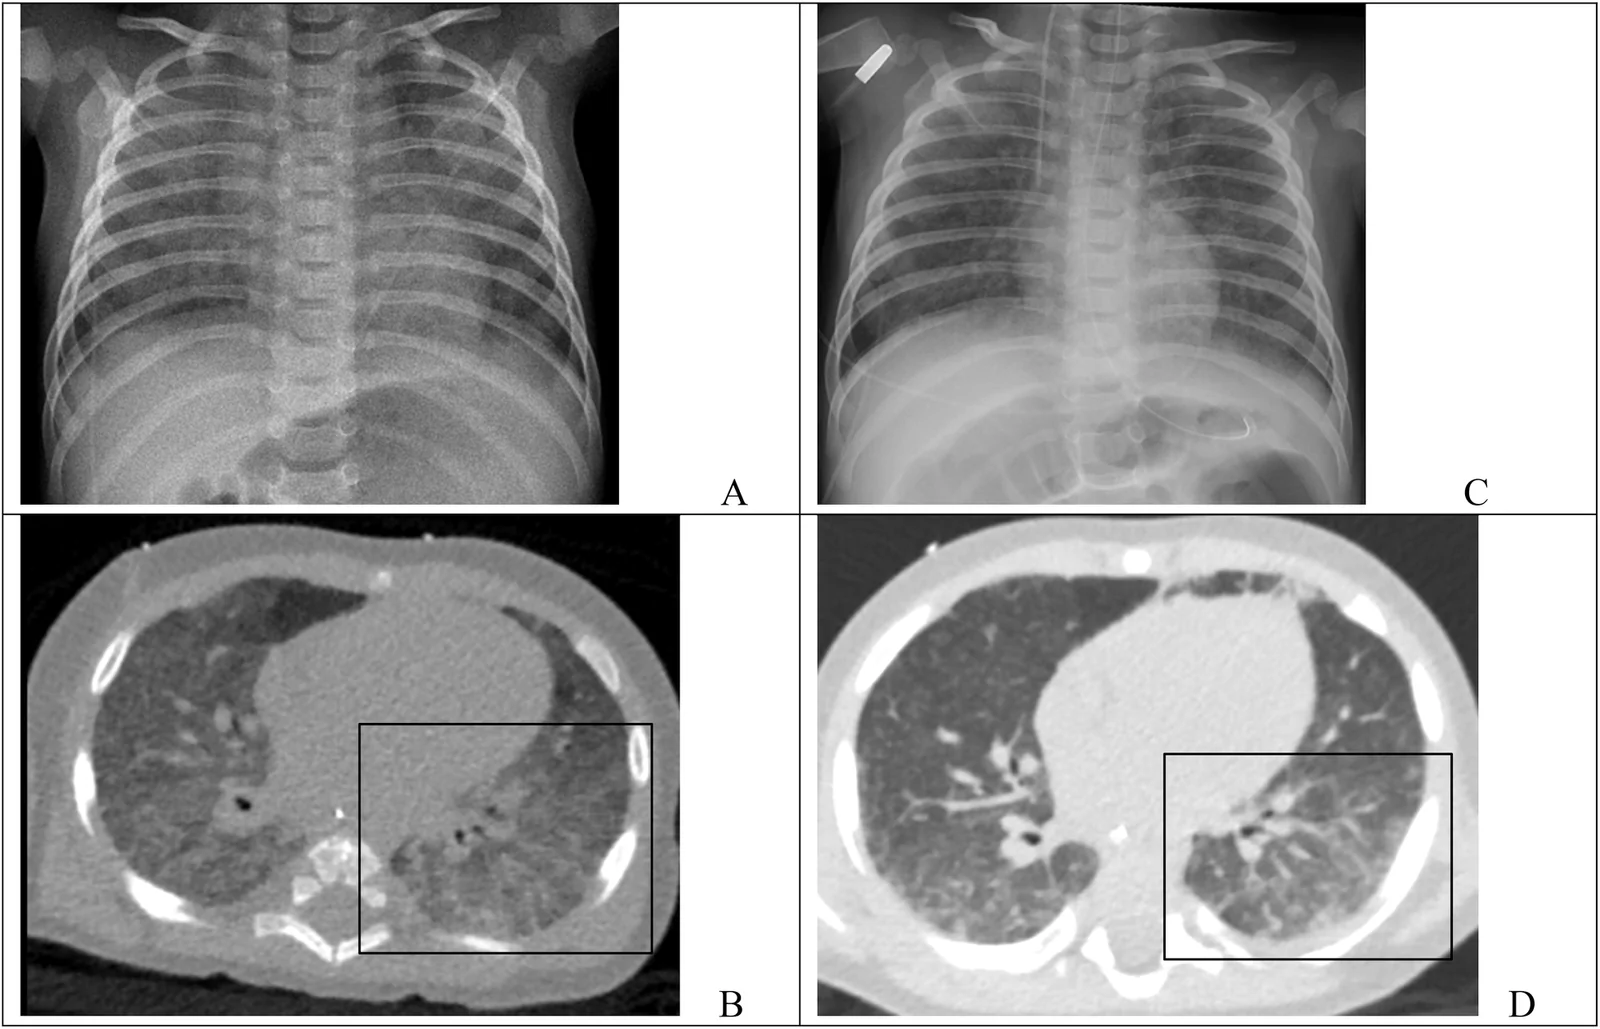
Chest imaging findings. (A) Initial chest radiograph (week 1): diffuse ground-glass opacities. (B) Initial chest CT (week 1): diffuse ground-glass opacities with interlobular septal thickening, predominantly in the basal regions. (C) Follow-up chest radiograph (week 6; 2 weeks post-treatment): near-complete normalization of the pulmonary parenchymal abnormalities. (D) Follow-up chest CT (week 6; 2 weeks post-treatment): marked improvement with residual basal ground-glass opacities, predominating in the dependent lung regions, and interstitial thickening.
